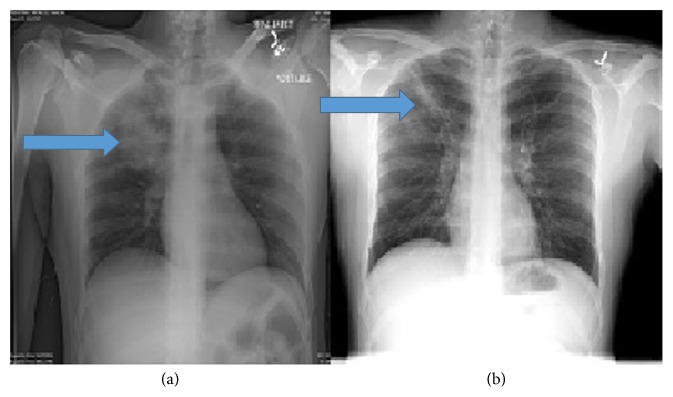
(a) Chest X-ray performed on admission, showing right upper lobe consolidation. (b) Chest X-ray performed at 2-month follow-up, showing right upper lobe scarring and improvement in consolidation.
